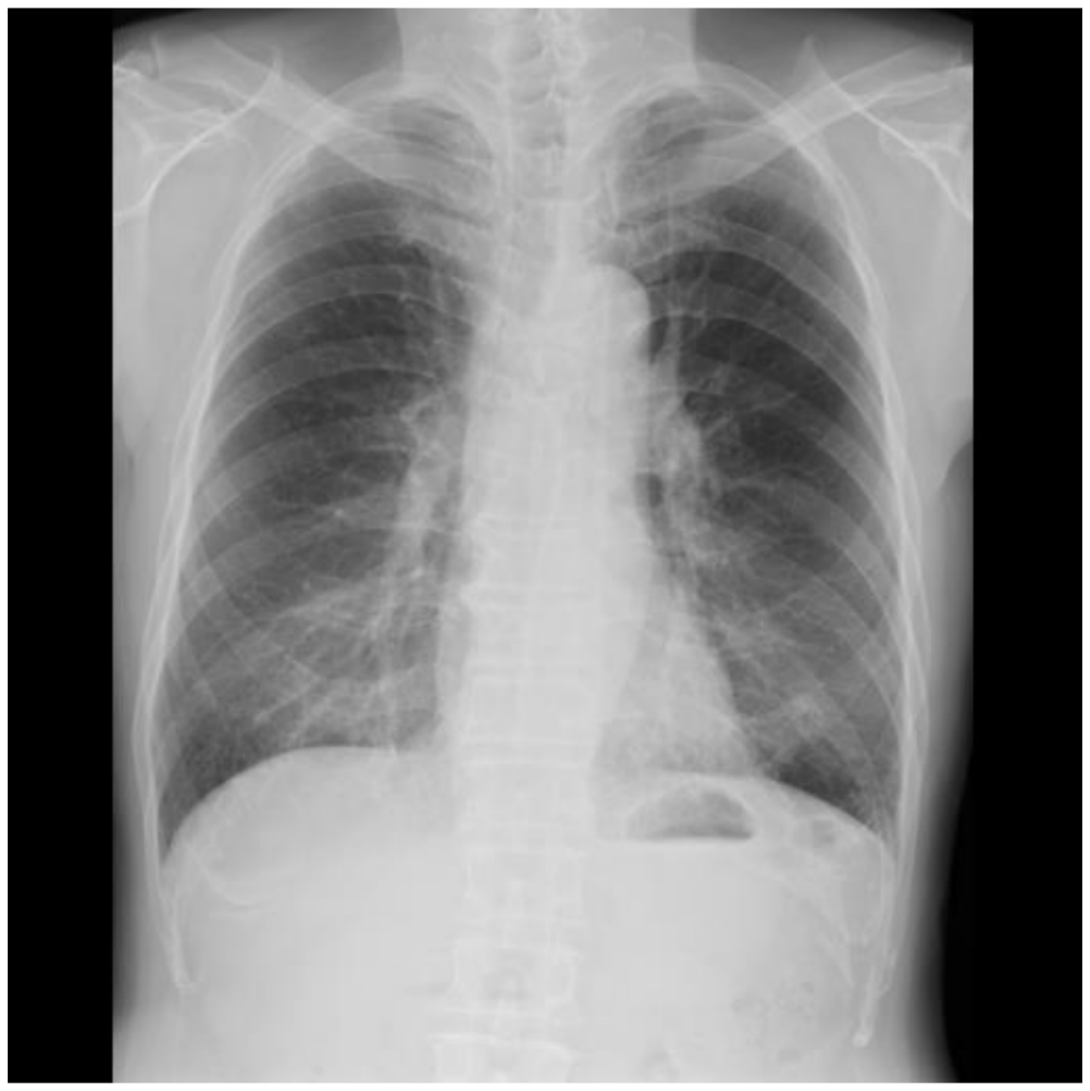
Sample chest radiographs used in this study. The original images were down-sampled and zero-padded to a 512 × 512 matrix with the aspect ratio preserved. Additionally, they were resampled to 224 × 224 and used as input.
